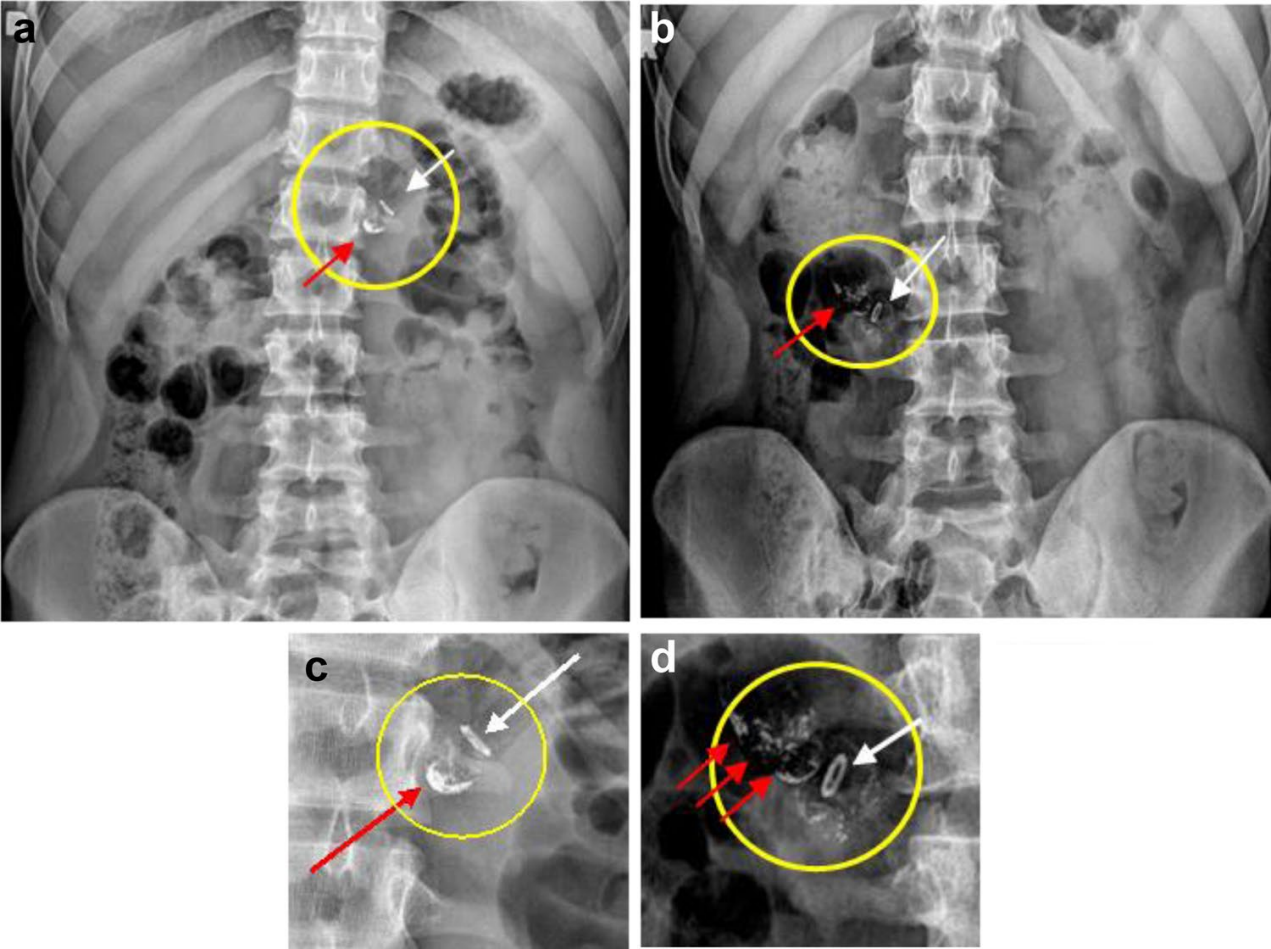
a Representative X-ray image of an intact RP residing in the stomach (encircled) showing a radio-opaque ring (which is part of the device) at one end of the device (white arrow) and barium sulfate powder inside the capsule shell at the other end (red arrow). b Representative X-ray image of a deployed RP in the small intestine (encircled). The radio-opaque ring (white arrow) is part of the device whereas barium sulfate is dispersed inside the intestinal lumen (red arrows). c Magnified encircled area from a. d Magnified encircled area from b.
